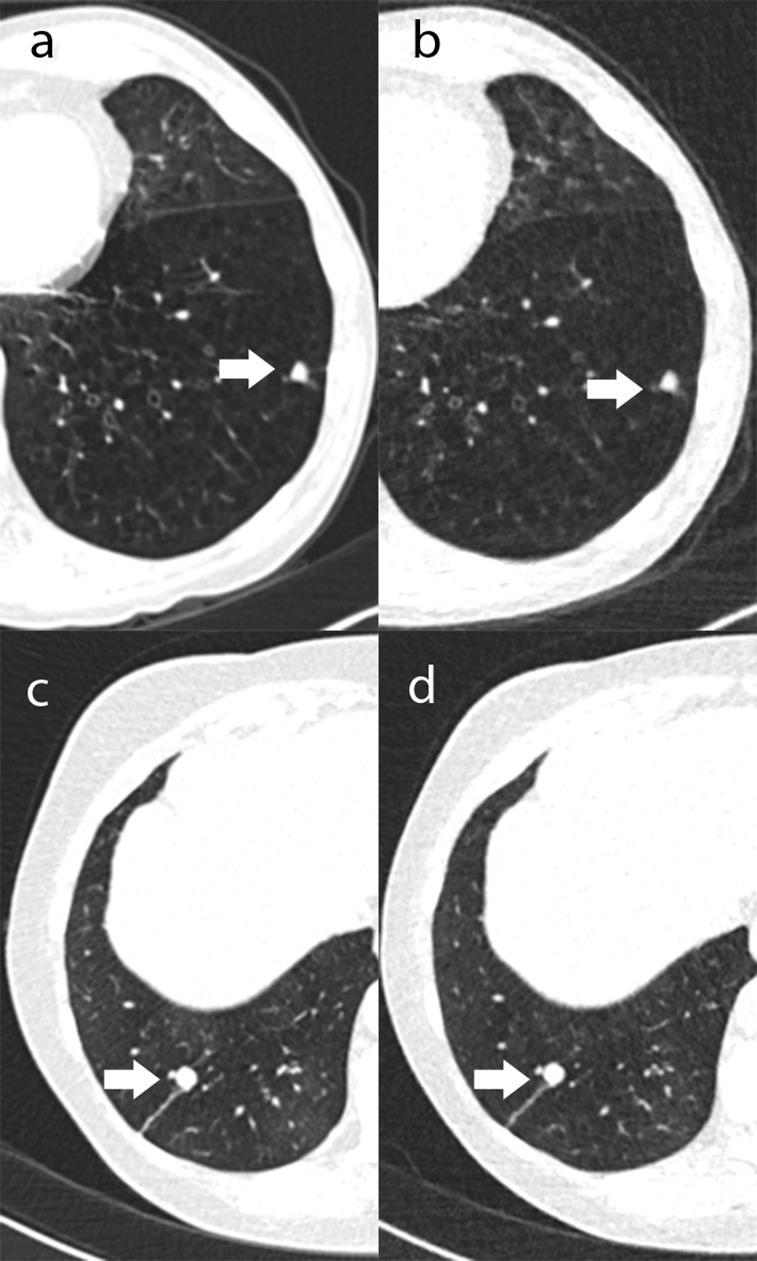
Transverse thorax CT images with true-positive nodules found on both ULDCT and RDCT from two patients. (a) and (b) are RDCT and ULDCT, respectively. (c) and (d) are RDCT and ULDCT, respectively. RDCT, response-dose CT; ULDCT, ultralow-dose CT.
